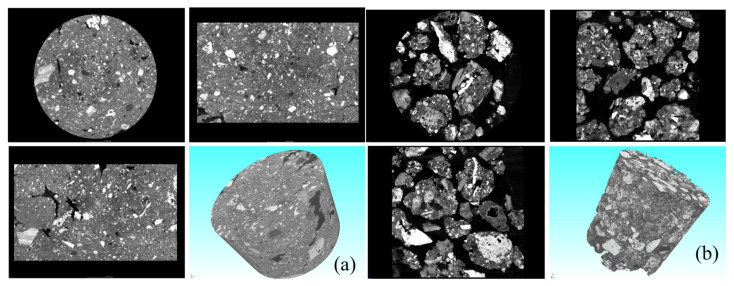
(a) Ordinary RM; (b) low-alkalinity RM CT scan quadrature view.
